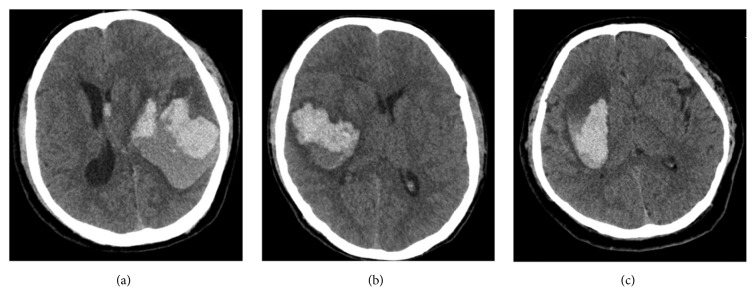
Classic cranial CT scans of Blend Sign I. The whole hematoma was composed of low-density and high-density hematomas with a clear boundary, The difference in the CT measurement value was higher than 18 HU.
